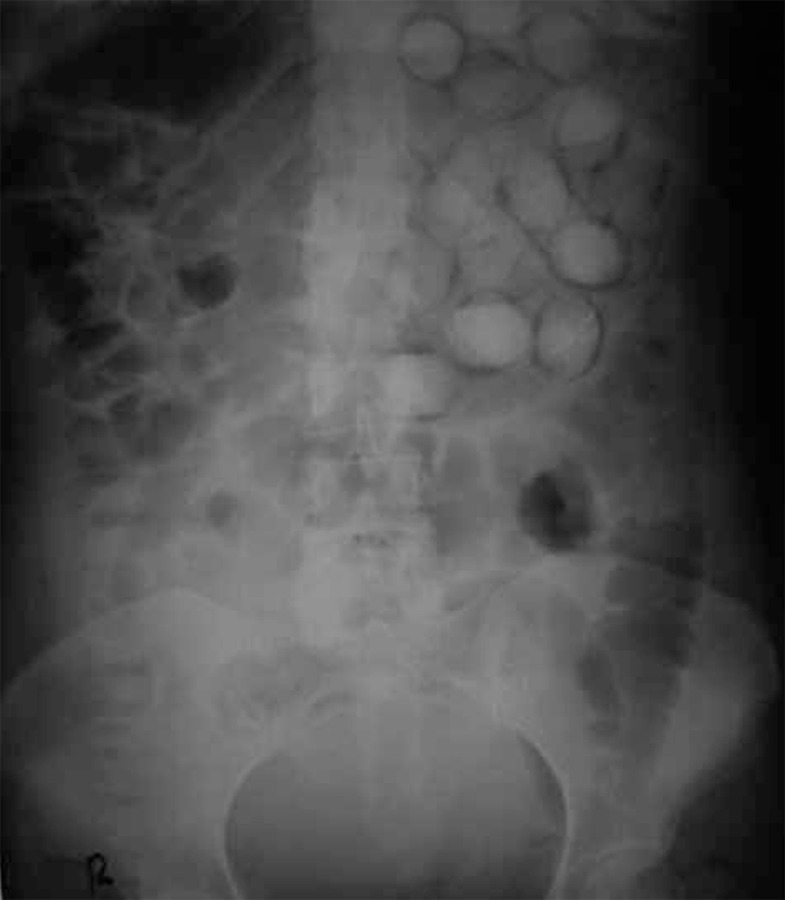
Abdominal x-ray (the same patient as Figure 1) reveals multiple, oval radiopaque packets throughout the abdomen.
